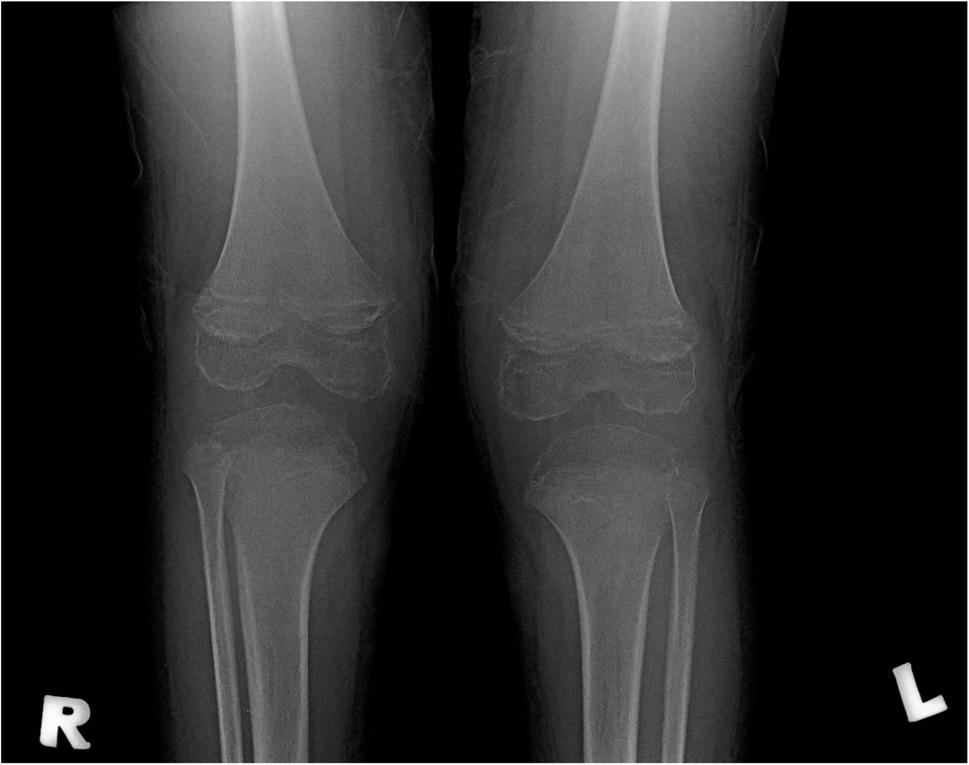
Bilateral knees radiographs show osteopenia and epiphyseal enlargement of bilateral femurs and tibias
